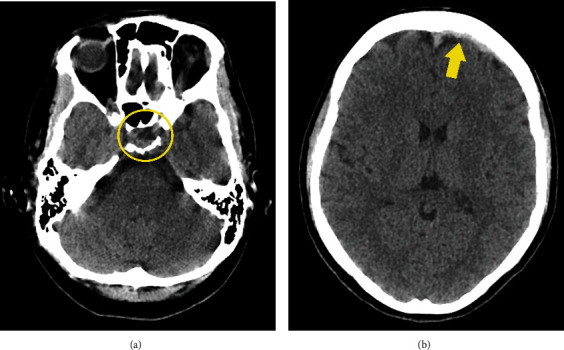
(a) Axial CT of pituitary apoplexy appearance indicates heterogeneous hyperattenuating intrasellar lesion due to the presence of hemorrhagic components with a fluid debris level (circle). (b) Axial CT image shows a thin layer of acute subdural hematoma in the left frontal region (arrow).
